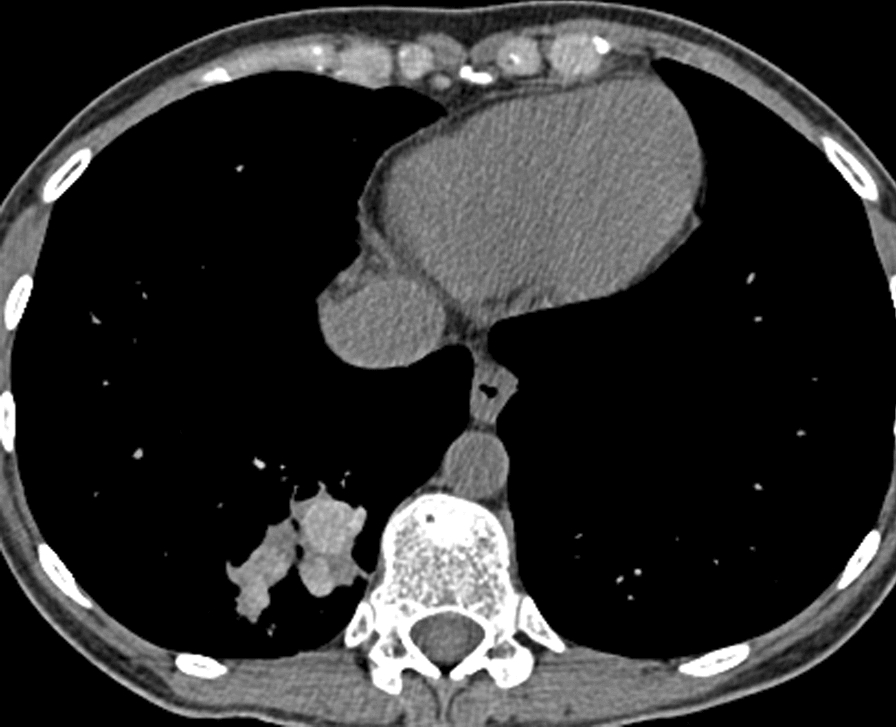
Hyper-attenuated mucous obstructing distal segments of the right lower lobe in ABPA. Woman with asthma who developed ABPA after exposure to a mouldy house (new renovation that leaked). During pregnancy starting coughing black plugs up and had an episode of ‘pneumonia’. CT scan shows rounded opacities posteriorly in the right lung base which are enlarged bronchi full of mucus. On the lung windows, rounded shadows are typical of mucus in bronchi, with some surrounding inflammatory change, on the bone windows (shown here), the mucus in some places appears white than others, consistent with hyper-attenuated mucous (HAM)
